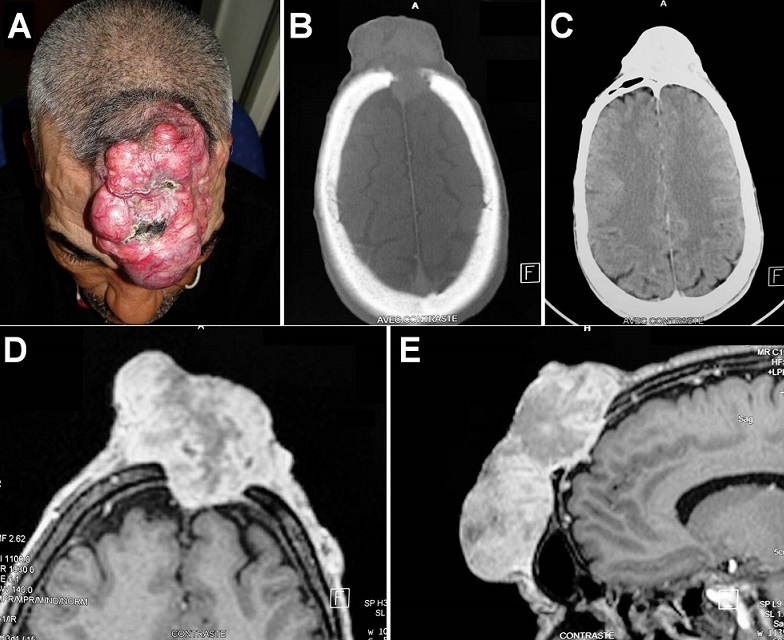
Patient photograph showing the midfrontal mass of the scalp (A). Axial cranial CT-scan revealing the frontal calvarial defect with poorly bony defined margins (B-C). Axial and sagittal T1-weighted MRI after gadolinium injection showing the enhanced soft heterogeneous extracranial process which invaded the dura and the superior longitudinal sinus (D-E)
